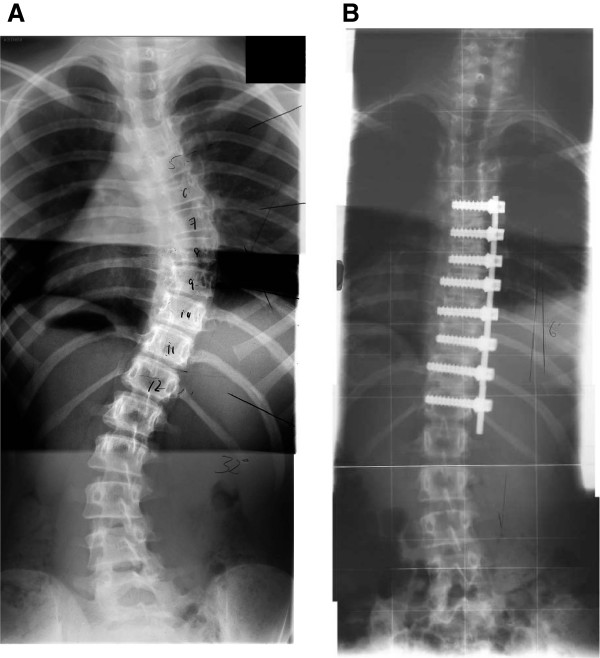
Radiographs of an AIS patient’s spine, pre-operatively (A) and post-operatively (B) after undergoing a single rod, anterior procedure.
